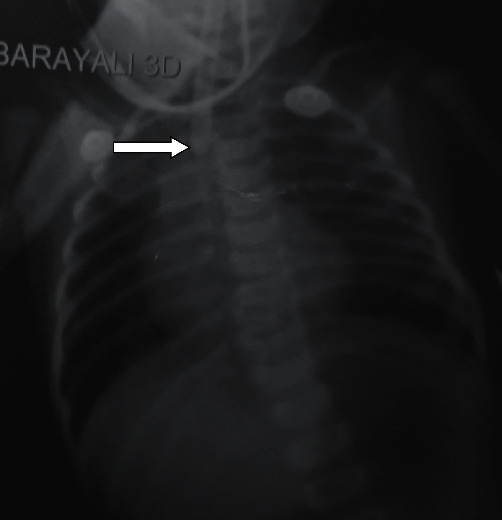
X-ray chest prior to the TEF repair showed the red rubber catheter (white arrow) in the proximal esophagus with presence of gas in the stomach and intestine consistent with TEF.
